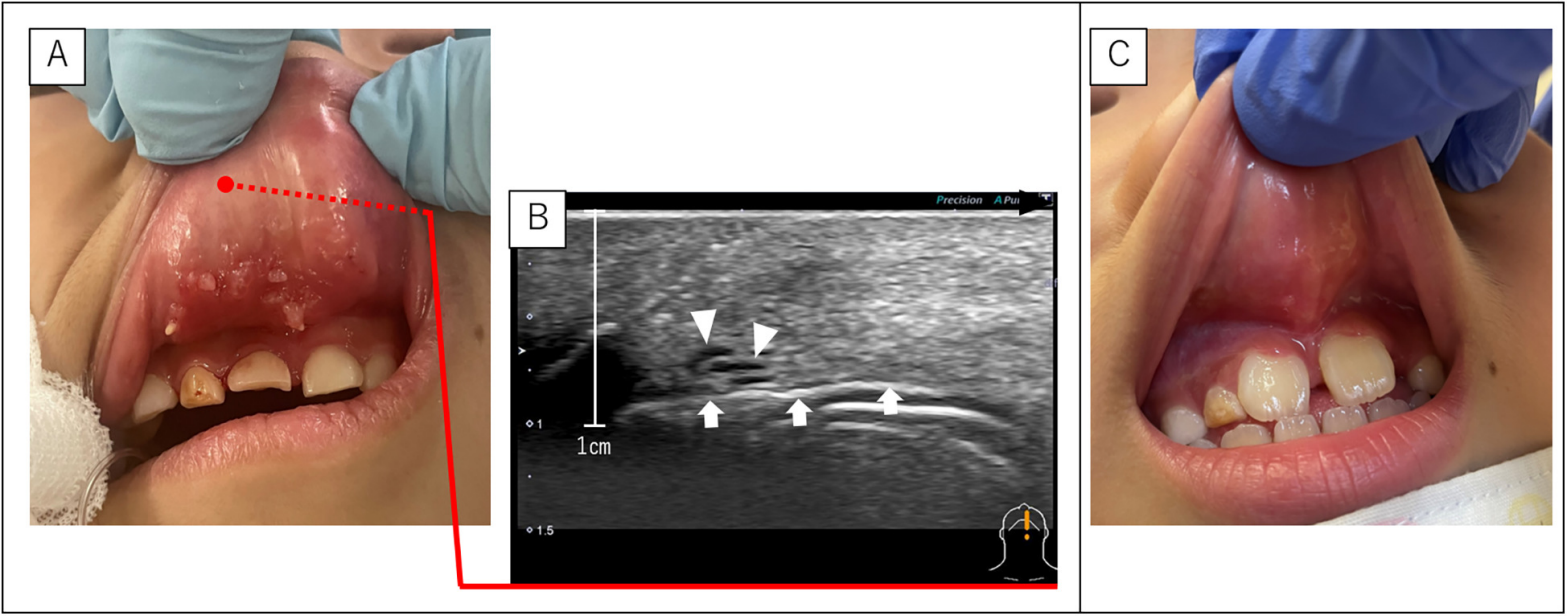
SLM and LM of case 6. (A) Pre-sclerotherapy findings showing SLM on the back of the upper lip. (B) Ultrasonography (sagittal section) showing a fine LM on the upper lip closest to the nose. The black arrowhead indicates the direction of the lip edge (caudal side). White arrowheads indicate the LM. White arrow indicates air in the oral cavity. (C) Post-sclerotherapy findings show that the SLM is almost unidentifiable. LM, lymphatic malformations; SLM, superficial lymphatic malformation.
